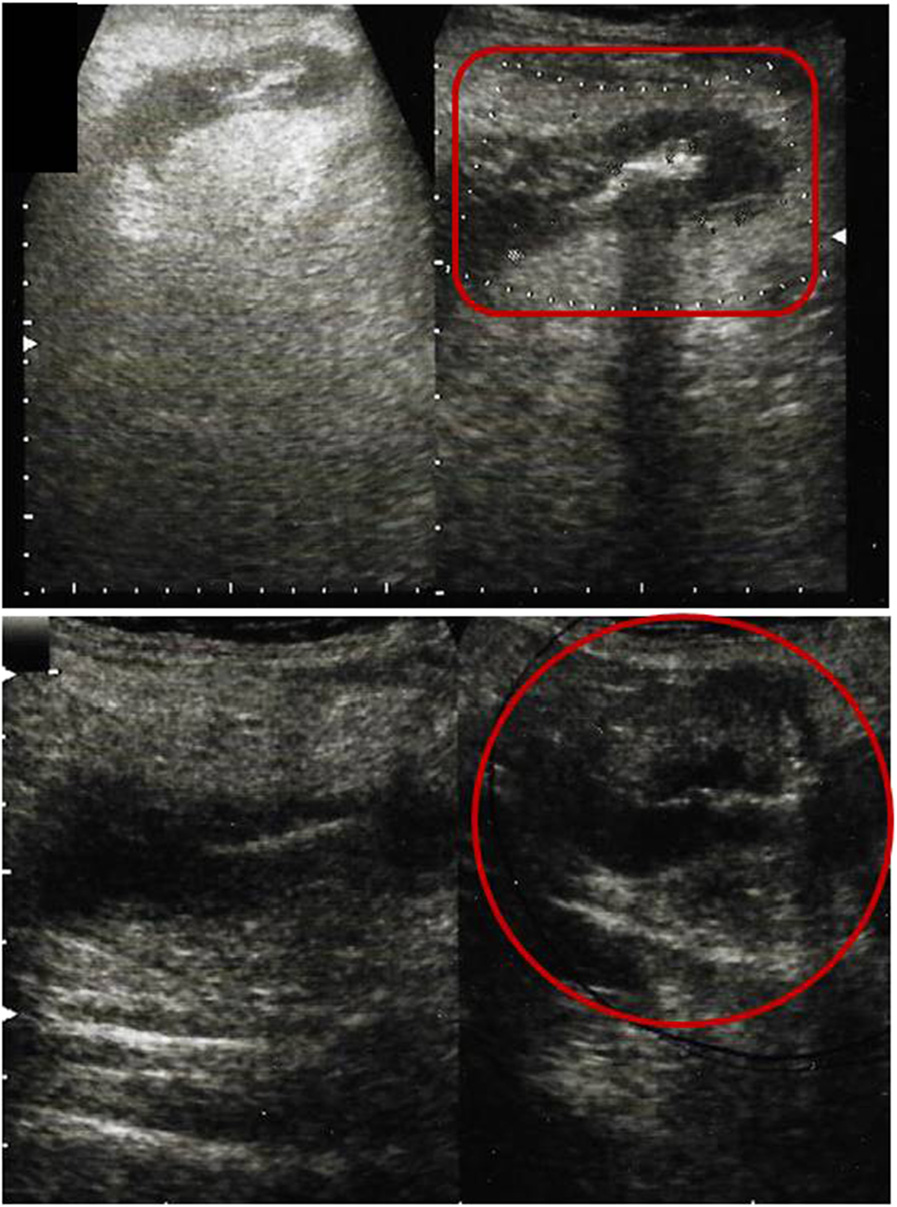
Ultrasonography revealed an inflamed tubular structure inside the right inguinal canal. This tubular structure looked similar to the appendix and was extending along the inguinal canal demonstrating the decision of emergency surgery treatment.
